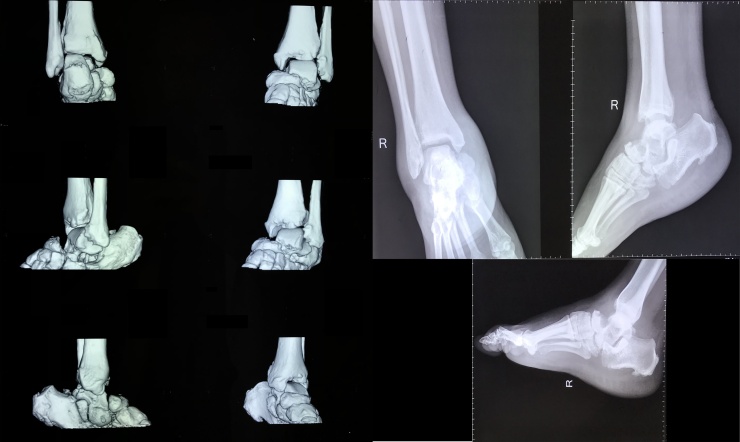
Radiographic result of the right foot demonstrated a deformity of the forefoot bone.
